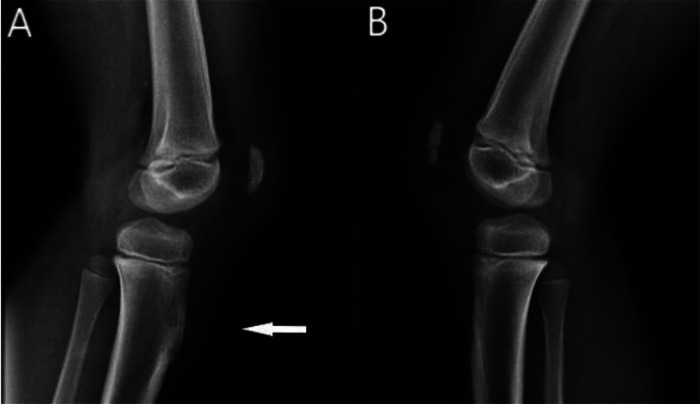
Lateral view knee X-ray. Lef knee (A) showed a bony bump of the tibial tuberosity (arrow). Right knee (B) was completely normal.
